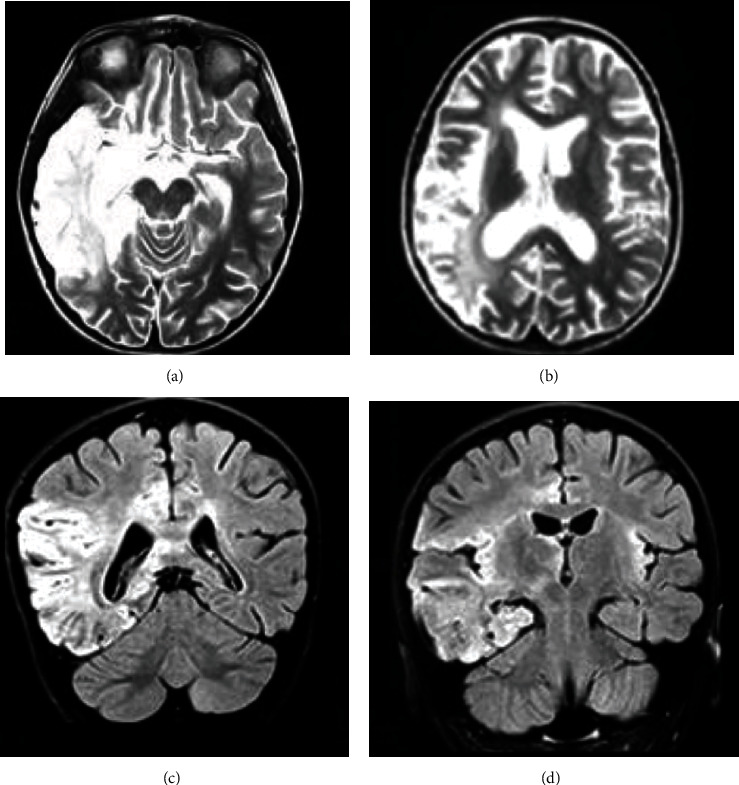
Brain magnetic resonance imaging from a patient with post-HSV-1 autoimmune encephalitis and NMDAR antibodies showed corticosubcortical hyperintensity in the right temporoparietal lobe on T2 weighted (a, b) and fluid attenuation recovery (FLAIR) sequences (c, d).
